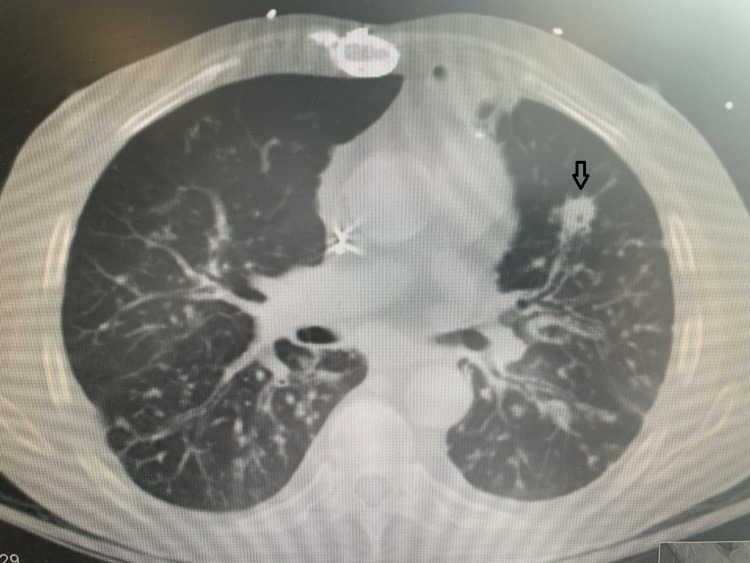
CT chest without contrast The arrow shows extensive patchy, and tree-in-bud opacities with a few of them demonstrating internal cavitation
